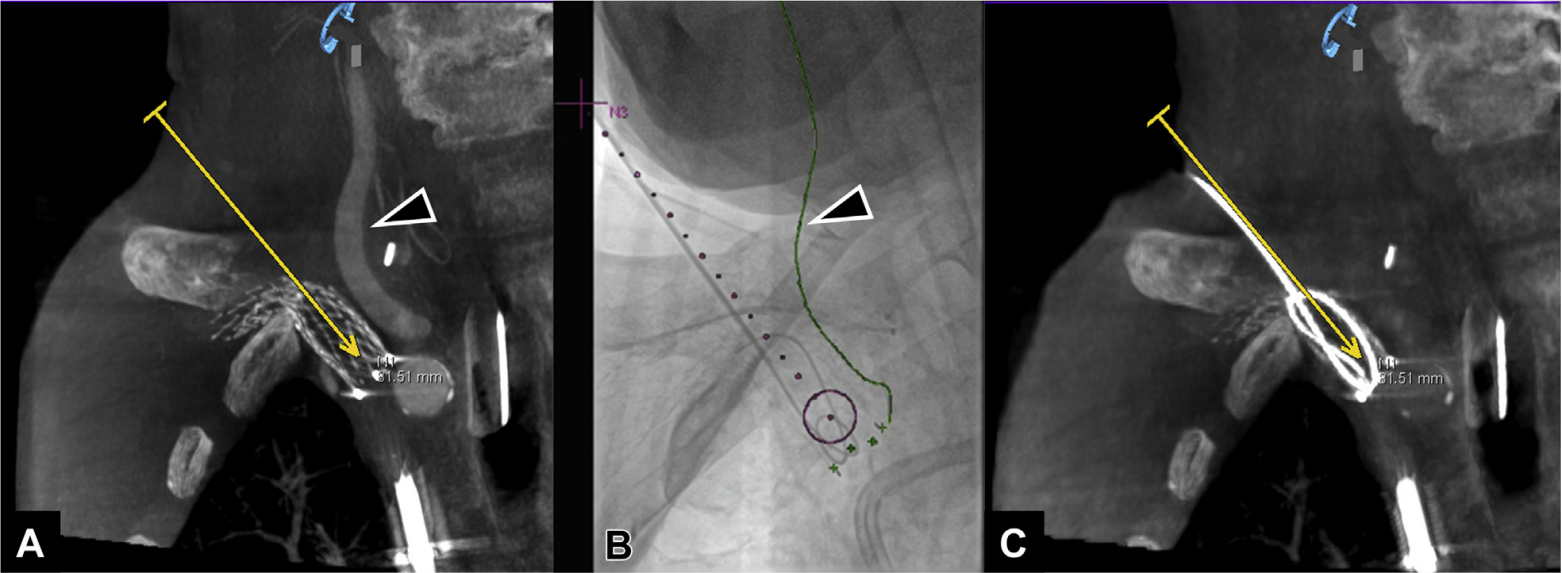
Cone-Beam Computed Tomography Before and After Percutaneous Recanalization of the Occluded Stent (A) Coronal reconstruction of intraoperative cone-beam computed tomography images, showing the planned virtual needle path (yellow line) and its relationship to the right subclavian artery and the right common carotid artery (arrowhead). (B) Insertion of an 18-gauge needle along the virtual needle path from cone-beam computed tomography overlaid on 2-dimensional fluoroscopy (purple dotted line). The purple circle indicates the target site inside the occluded stent. The green line indicates the lateral margin of the right common carotid artery (denoted by the arrowhead). (C) Coronal reconstruction of postrecanalization noncontrast cone-beam computed tomography confirming the location of the guidewire inside the occluded stent along the planned virtual needle path (yellow line). N1 = needle path 1; N3 = needle path 3.
